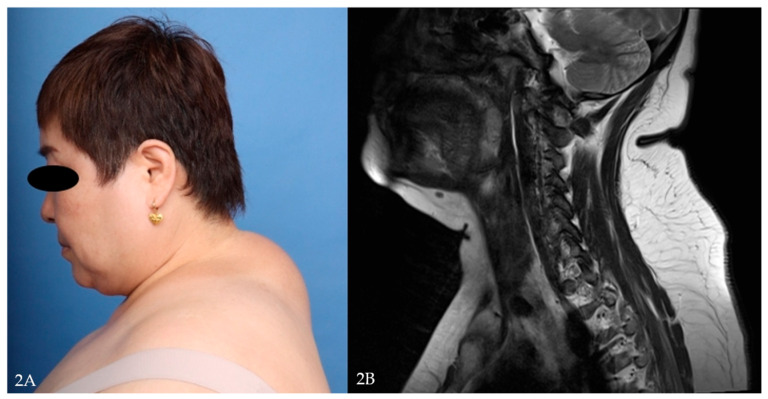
(A) Preoperative clinical photograph showing prominent buffalo hump. (B) Sagittal T2-weighted MRI demonstrating diffuse subcutaneous fat accumulation in the cervicodorsal region.
